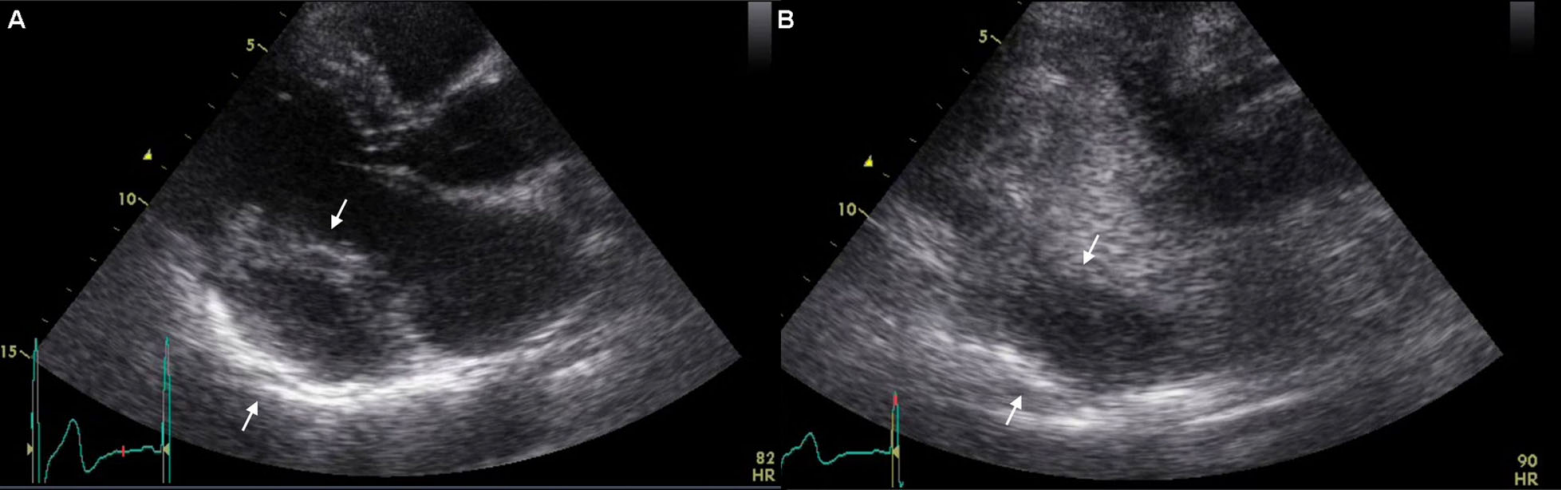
Transthoracic echocardiography. Panel A depicts long axis parasternal view of the hydatic cyst at the posterior wall of the heart (arrows). Panel B shows the same view using contrast media depicting the clear boarders of the cyst, without blood perfusion in the area of interest (arrows).
